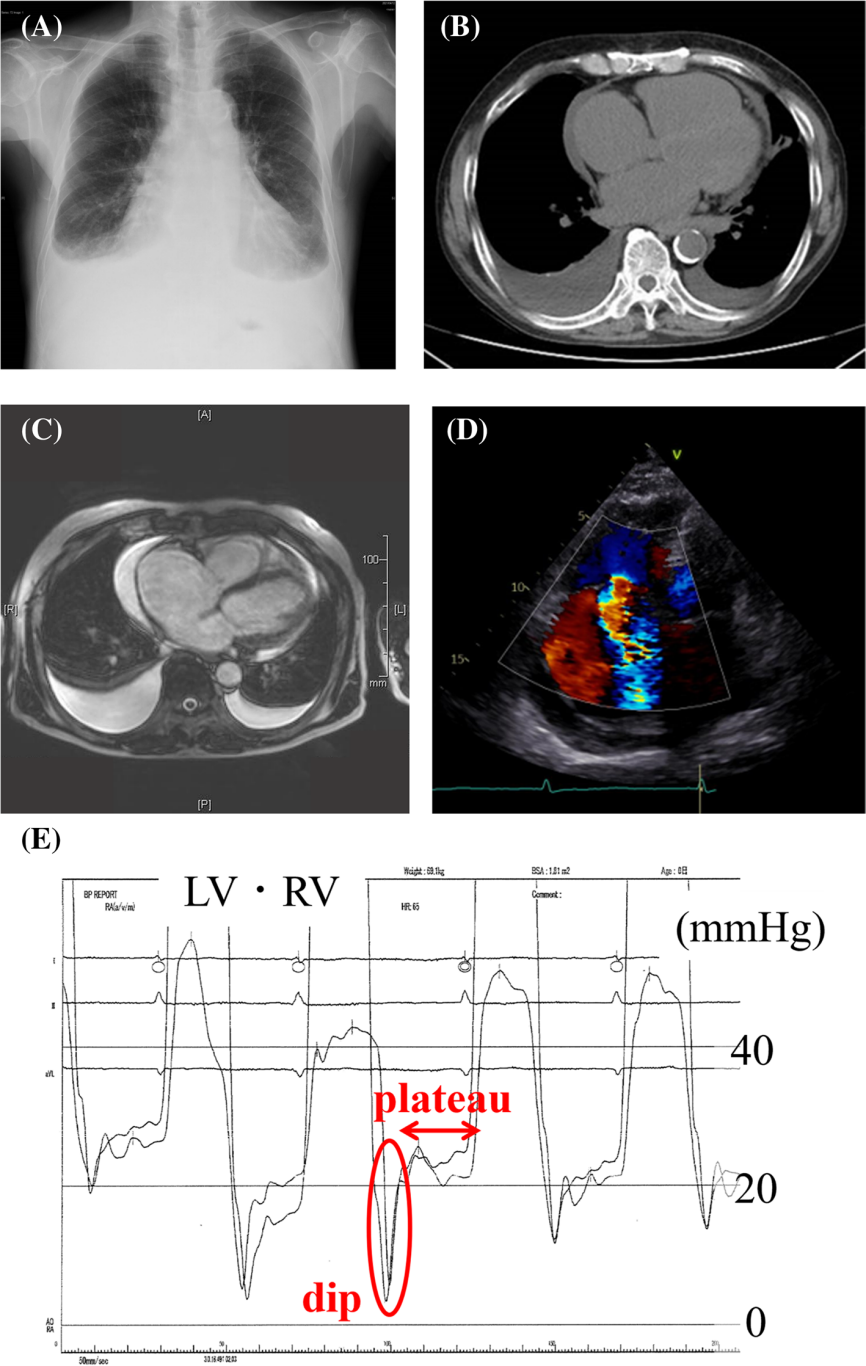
A Chest X-ray showed cardiomegaly and bilateral pleural effusion. No calcification of the pericardium was observed. B Bilateral pleural effusion and a small amount of pericardial effusion were detected in computed tomography. No calcification or thickening of the pericardium was observed. C Cardiac magnetic resonance imaging (cine image) demonstrated no thickening of the pericardium, nor adhesion between myocardium and pericardium. D Trans-thoracic echocardiographic color Doppler image of apical four-chamber view showed solitary severe TR and enlarged bilateral atria. E The pressure waveforms obtained for both the right and left ventricles on right heart catheterization demonstrated the “dip and plateau” pattern typical of constrictive pericarditis
